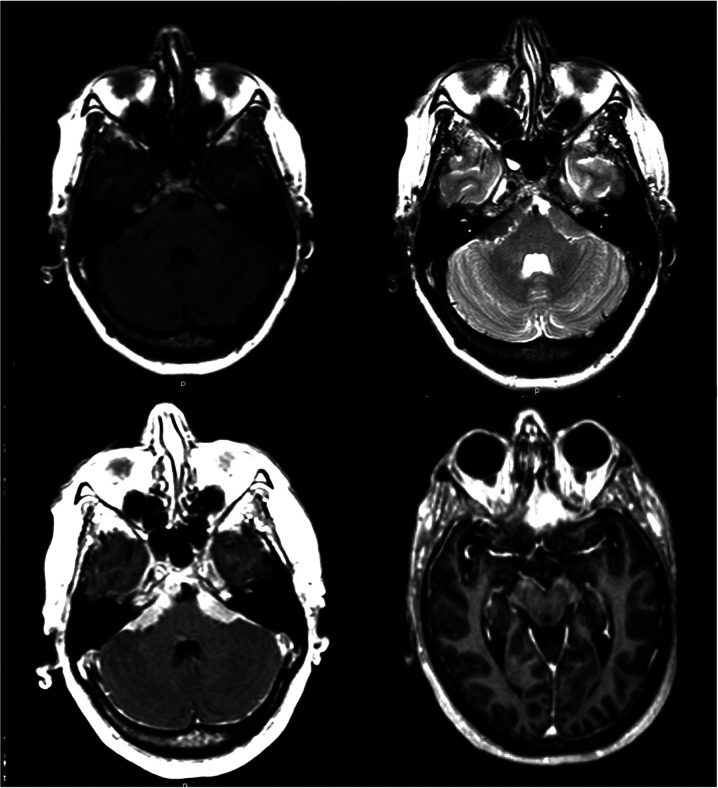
MRI sequences demonstrating typical appearance of extensive skull base meningioma (upper row left: T1 without contrast, upper row right: T2, lower row: T1 with contrast).
